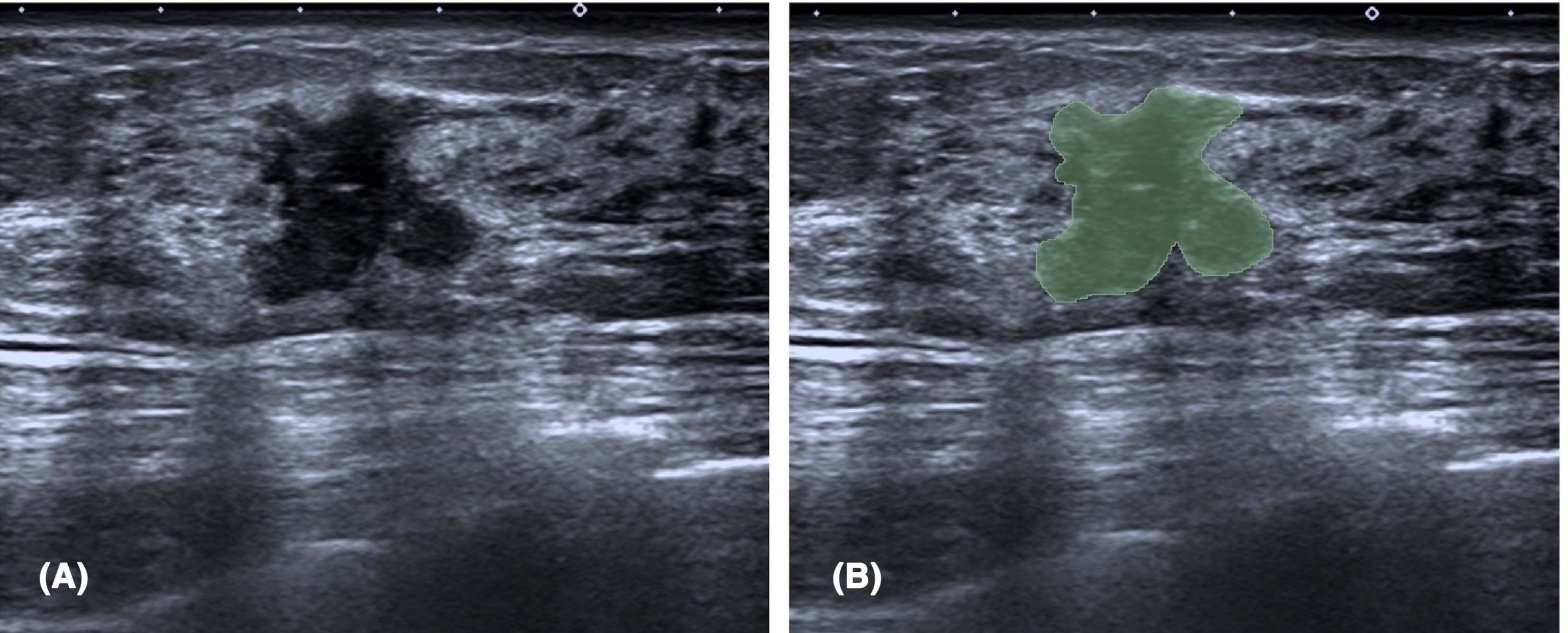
(A) 2D grayscale ultrasound image of breast lesion. (B) Manually outline the region of interest using software 3D slicer.
